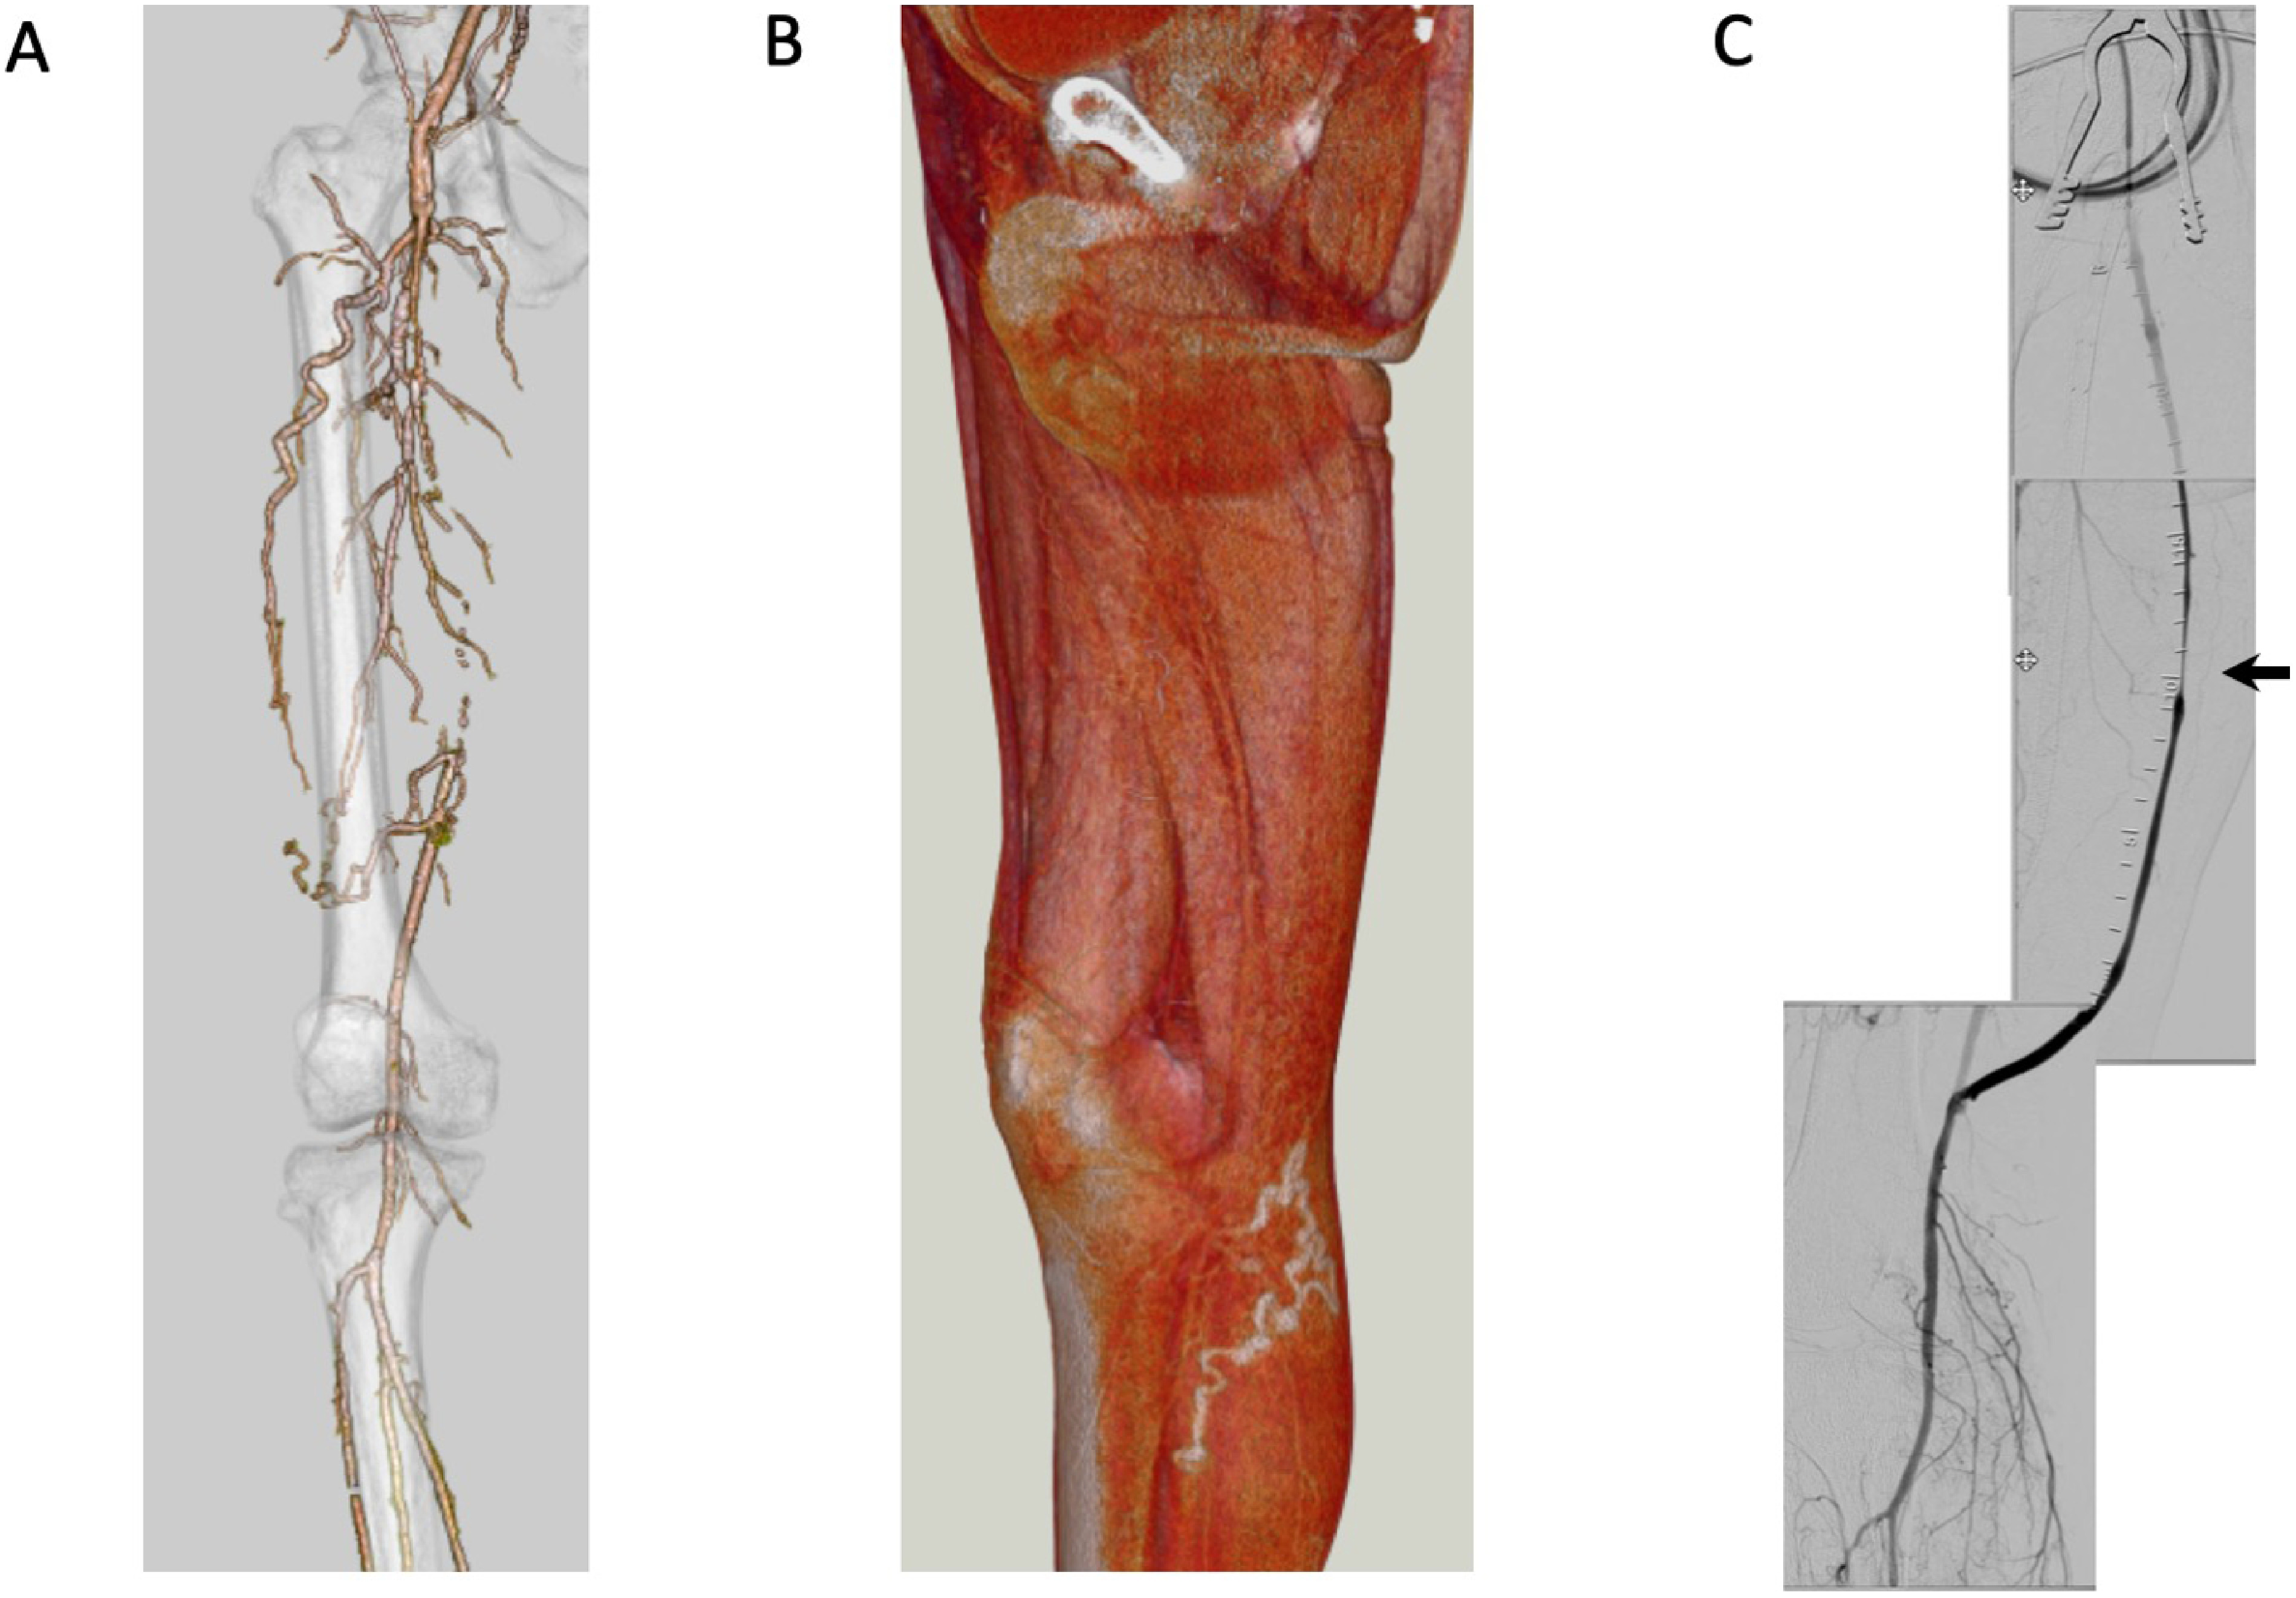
Fig. 2 Case 2. (A) Preoperative computed tomography angiography revealed that the superficial femoral artery was occluded. (B) Preoperative computed tomography venography revealed the great saphenous vein having no morphologically conspicuous abnormalities except for some segmental type varicosity at the crus and some sinusoid dilatations at the origin of valves. (C) Completion angiography revealed that the right femoropopliteal bypass was patent. Vasospasm was observed in the midportion of the vein graft (arrows).
